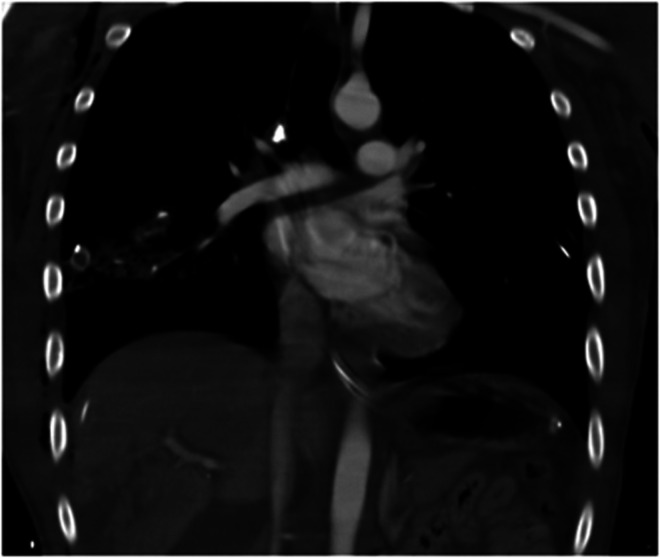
CT chest showing trauma to the right lung with a chest tube in place. Additionally there is a tract from the bullet in communication with the pulmonary vasculature.
